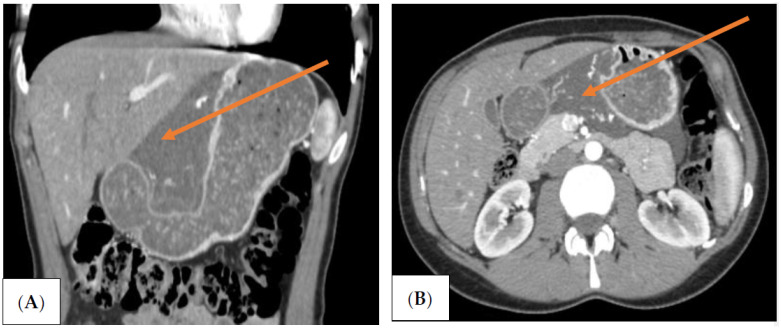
(A,B) Computed tomography (CT) image of the abdominal cavity. There is a visible mass located at the lesser curvature of the stomach (orange arrows).
